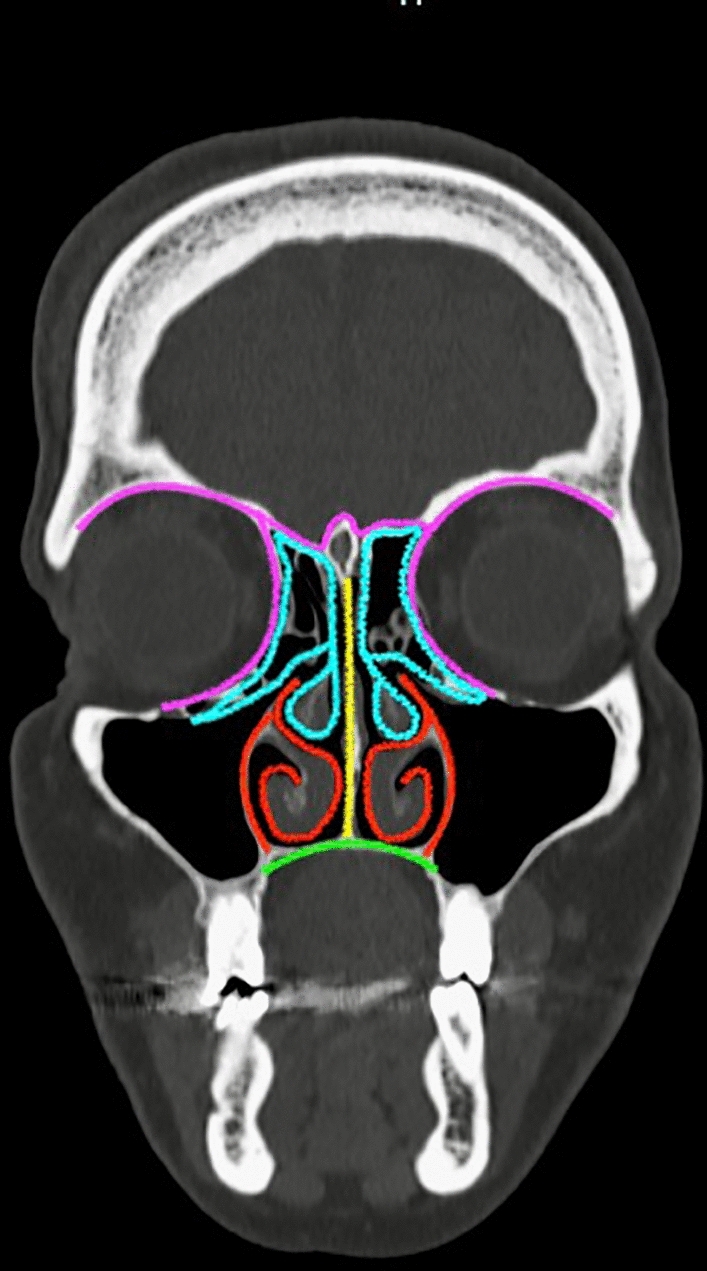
Graphical depiction of the prevalence of identified cocaine-induced midline lesions according to their location. A non-CIMDL patient coronal computed tomography image is used as an anatomical reference. Yellow: grade 1 CIMDL region (nasal septum, 99.2% of patients); red: grade 2a CIMDL region (inferior turbinate and maxillary sinus medial wall, 59% of patients); green: grade 2b CIMDL region (palate, 29.9% of patients); blue: grade 3 CIMDL region (ethmoid bone, middle turbinate and superior turbinate, 22.8% of patients); purple, grade 4 CIMDL region (papyracea, orbit or skull base, 7.9% of patients)
